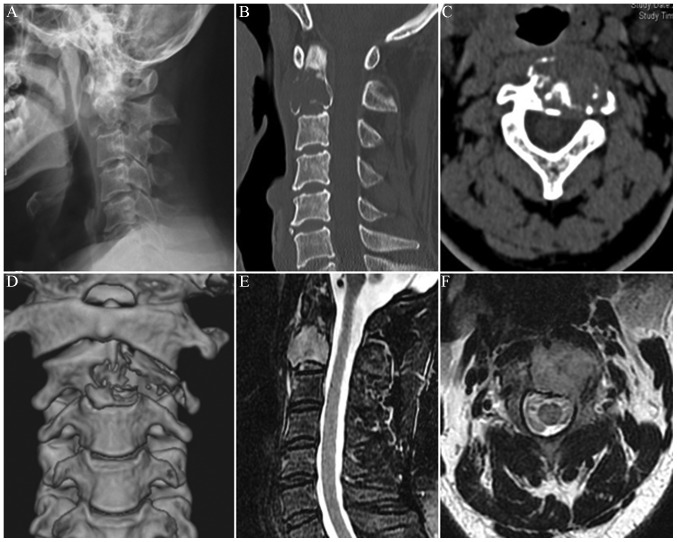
Series of images of the C2 metastasis. (A) Lateral X-ray shows a C2 destructive process, which was confirmed by (B and C) CT, (D) CT 3D and (E and F) MRI imaging. CT, computed tomography; 3D, three dimensional; MRI, magnetic resonance imaging.
